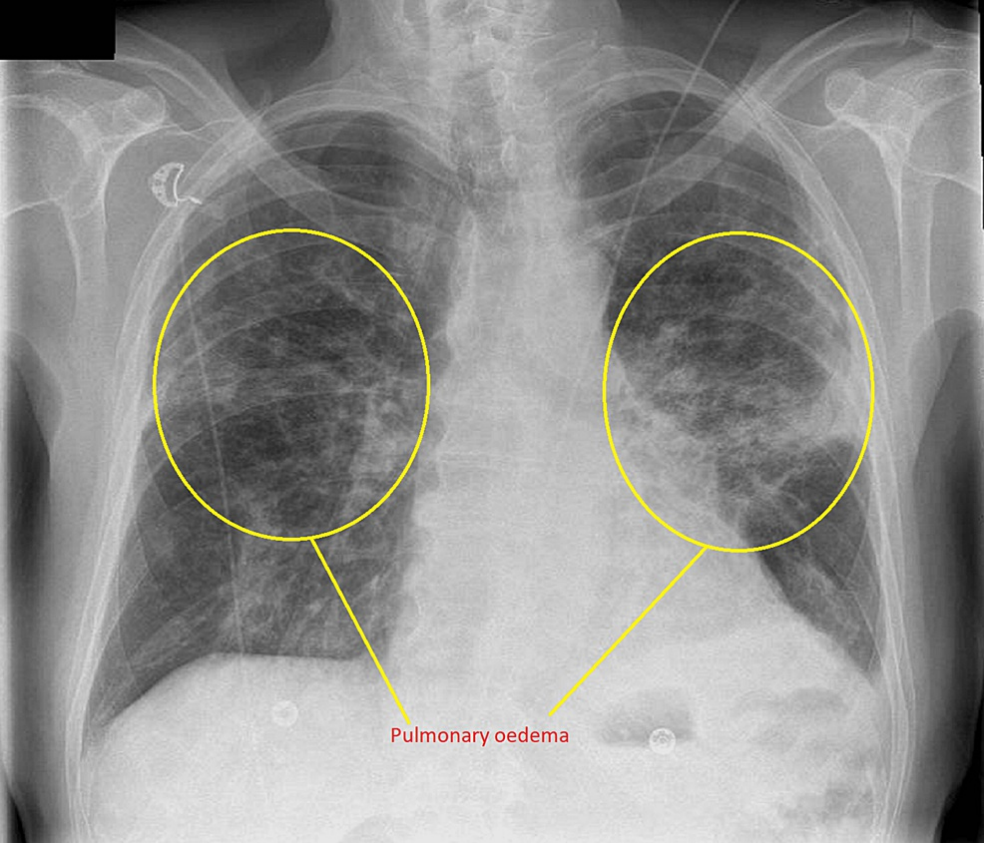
Chest X-ray. Pulmonary oedema significantly improved after the administration of intravenous diuretic.
